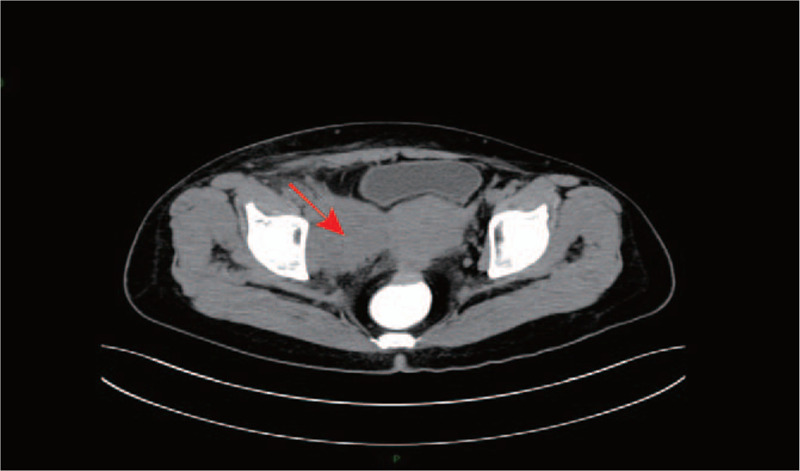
Plain and enhanced spiral CT scan of the pelvis showed soft tissue mass in the right adnexa, about 8.2 cm × 6.5 cm × 5.0 cm in size (shown by the red arrow).
